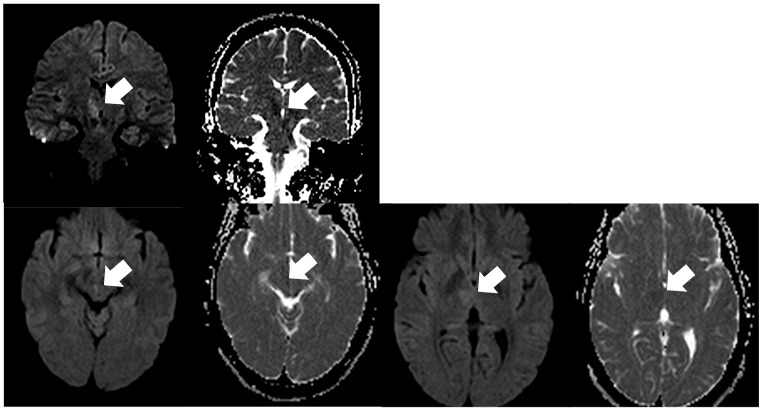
Acute ischemic lesion in the midbrain, specifically the medial longitudinal fasciculus, indicated by a white arrow. Upper row: coronal DWI (b = 1000 s/mm^2^) and corresponding ADC map; lower row: axial DWI (b = 1000 s/mm^2^) and corresponding ADC map. Lesion conspicuity is greater on coronal images compared with axial images.
